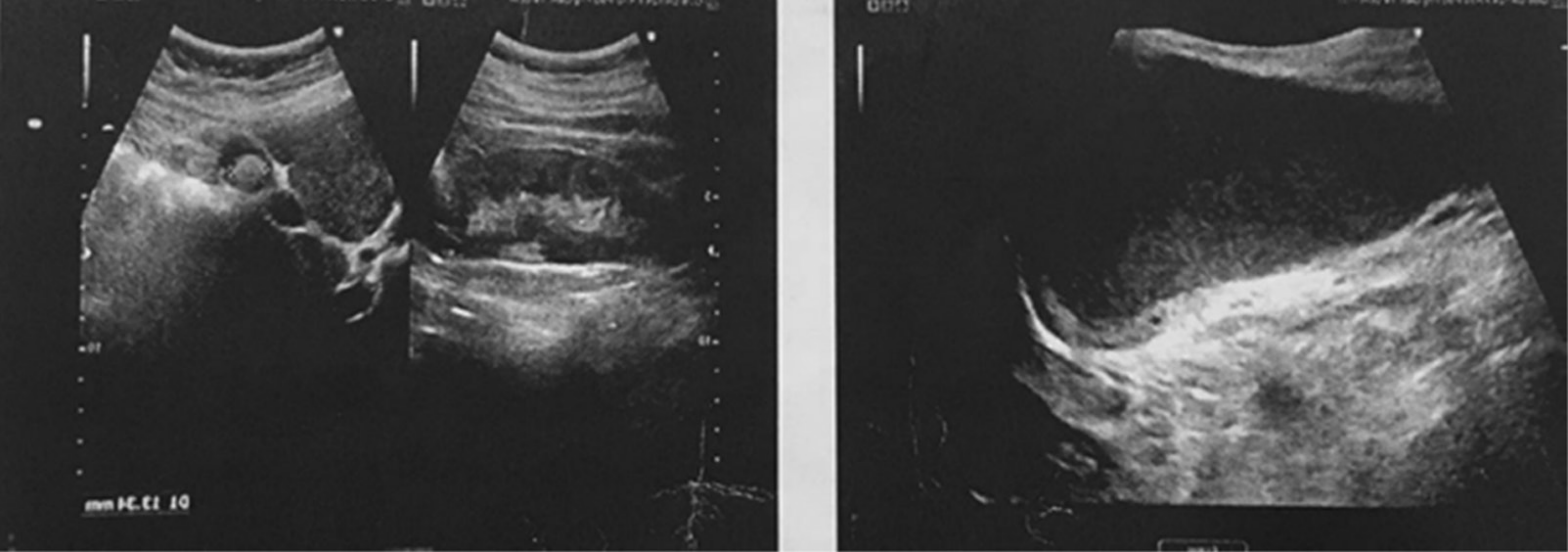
A mass measuring 90*44*55 mm in the right major labia spreading to the right inguinal on ultrasound.
